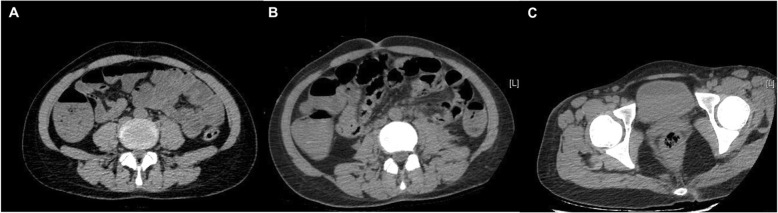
CT of the abdomen. a. The CT scan shows multiple air-fluid levels in the bowels suggestive of bowel obstruction on admission. b. The CT scan shows extensive free gases and fluids in the colon four hours after admission. c. The CT scan shows a high density mass in the bladder suggestive of cystorrhagia four hours after admission
